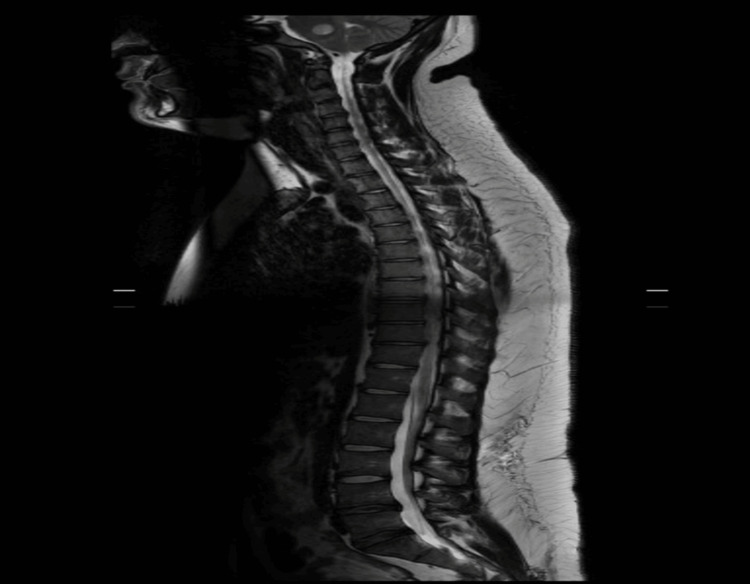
Sagittal Magnetic Resonance Imaging (MRI) of the Spine Sagittal MRI of the spine did not show evidence of leptomeningeal disease or drop metastasis.
